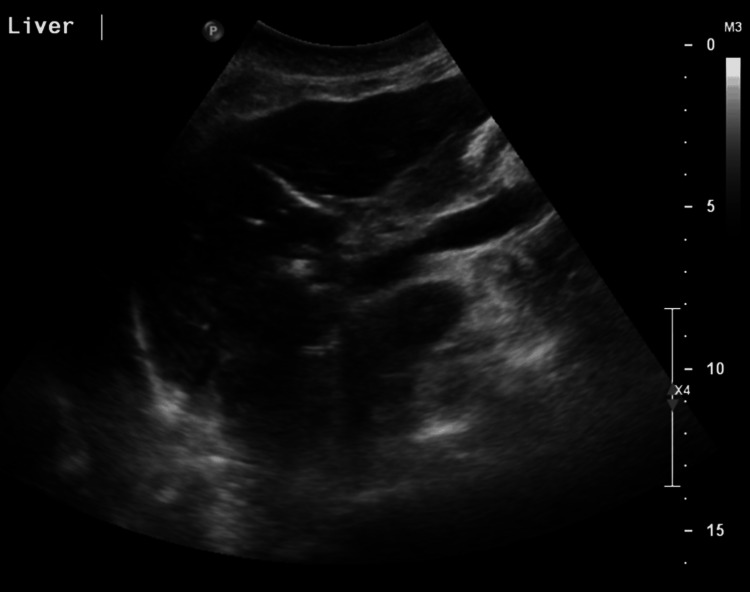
Ultrasound of the liver Ultrasound of the liver demonstrating the dilated external bile duct.
